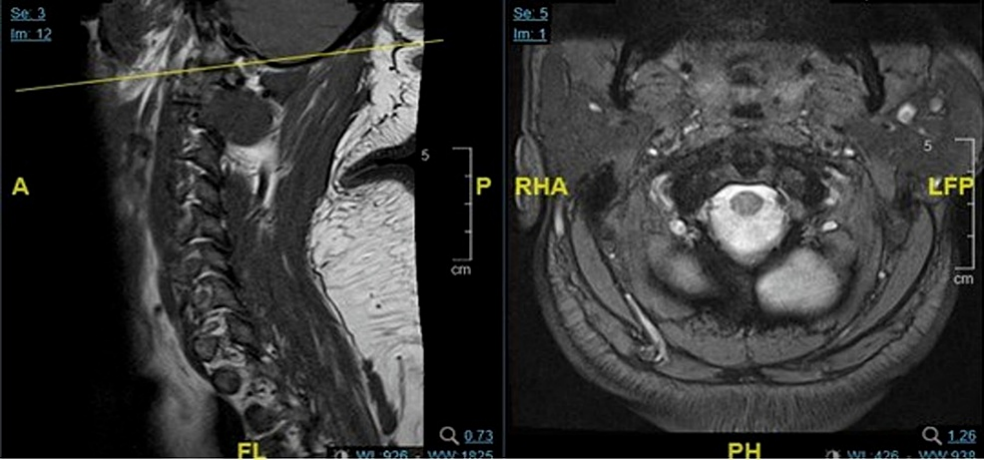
Magnetic resonance imaging (MRI) with no significant findings. A = anterior, P = posterior, FL= feet left, PH = posterior head, RHA = right head anterior, LFP= left feet posterior
